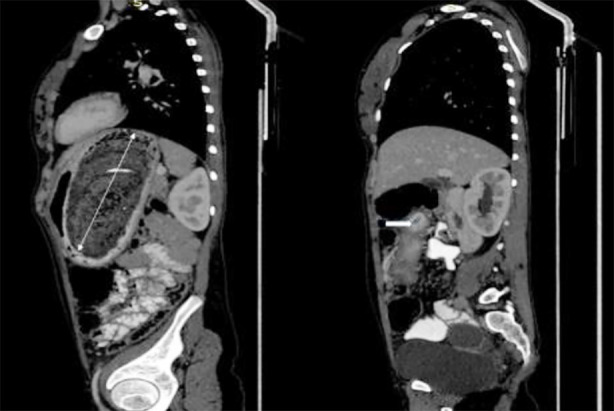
CT scan abdomen with contrast sagittal view, more prominent D2: showing a large heterogeneous intraluminal mass with a mottled gas pattern in the stomach, which extends through the pylorus up to the second part of the duodenum.
